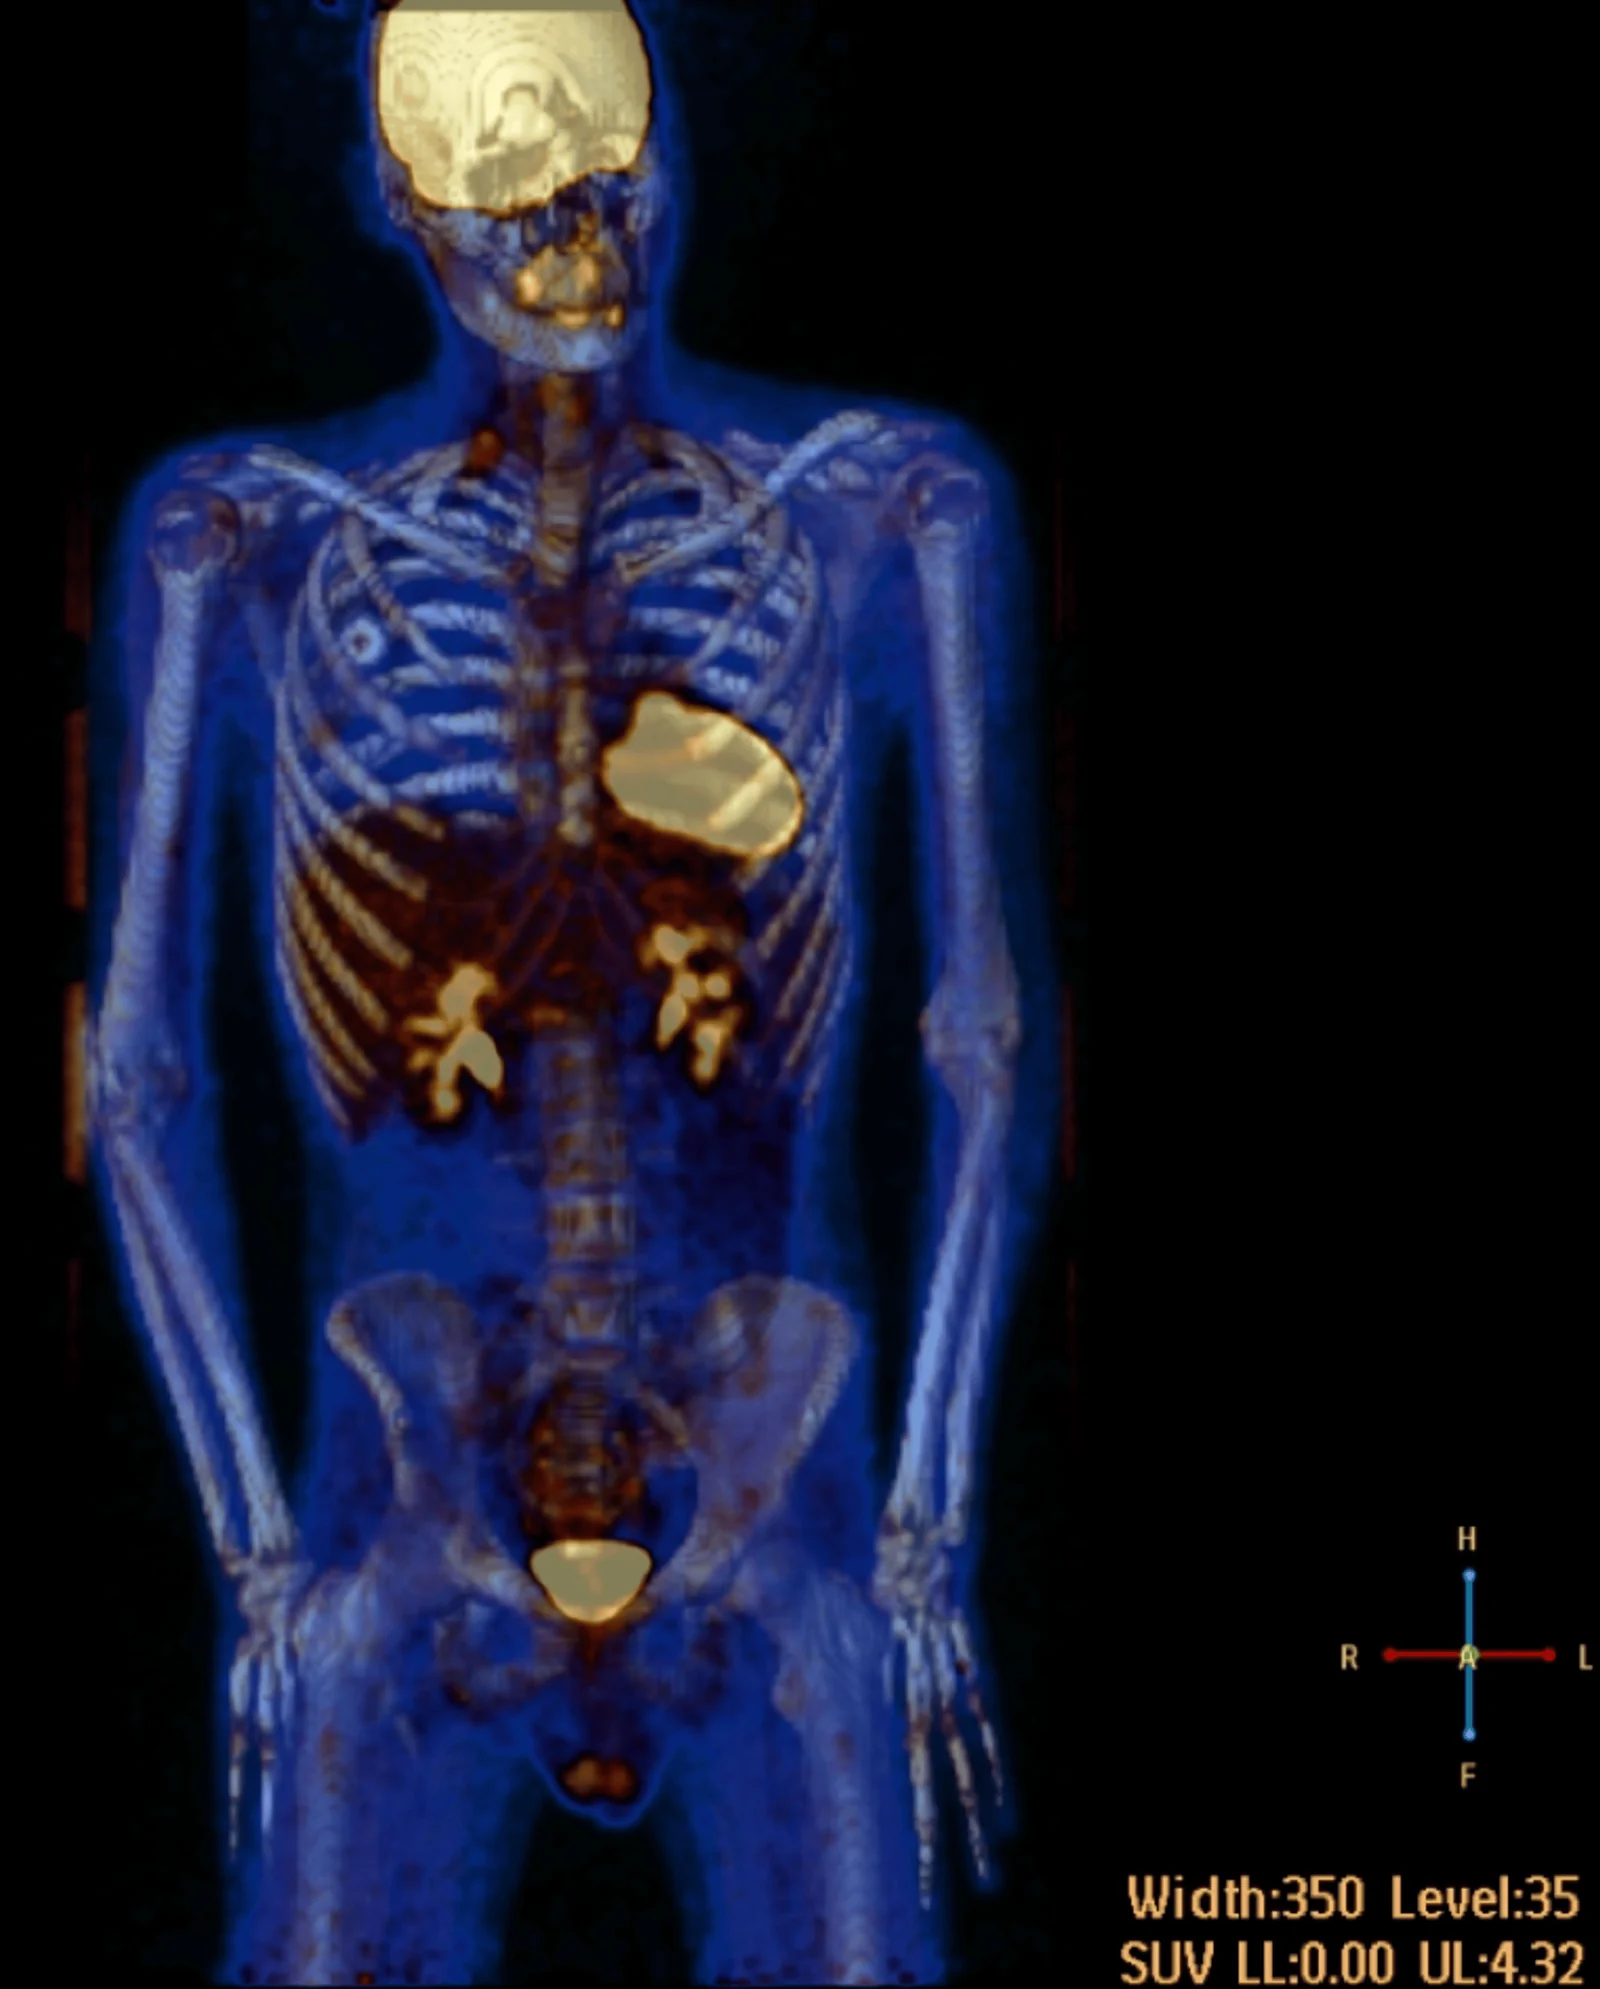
Three-dimensional reconstruction of the FDG PET/CT scan performed after the second BRESHAP cycle. A fluorodeoxyglucose (FDG) PET/CT scan was performed for lymphoma staging following the second cycle of BRESHAP (brentuximab vedotin, etoposide, methylprednisolone (Solu-Medrol), high-dose cytarabine (Ara-C), and cisplatin) chemotherapy. Physiologic FDG uptake was noted in the brain and myocardium, with normal tracer excretion via the renal collecting systems and urinary bladder (depicted in yellow on the color scale). No hypermetabolic foci suggestive of residual or active disease were identified. These findings are consistent with a complete metabolic response to the chemotherapy regimen.
